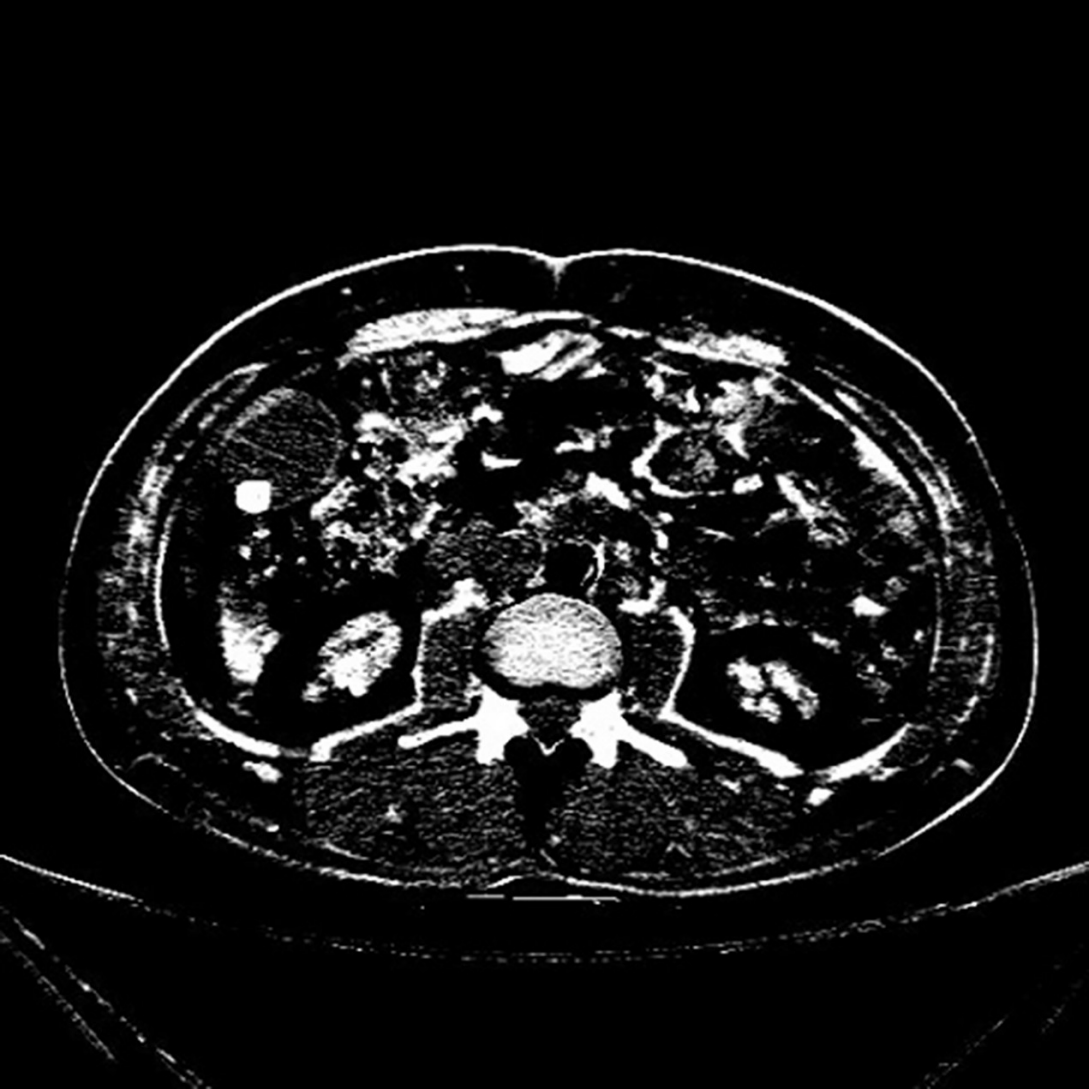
The CT image by histogram equalization.
